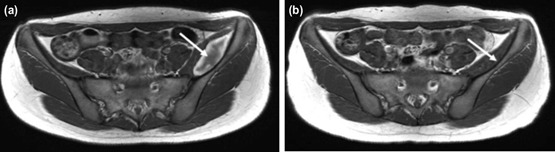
Magnetic resonance imaging scan showing the following findings: (a) Scan obtained 20 days post-injury showing an iliacus hematoma measuring 66×22mm in diameter (white arrow). (b) Scan obtained 80 days post-injury showing near-complete disappearance of the iliacus hematoma (white arrow).
